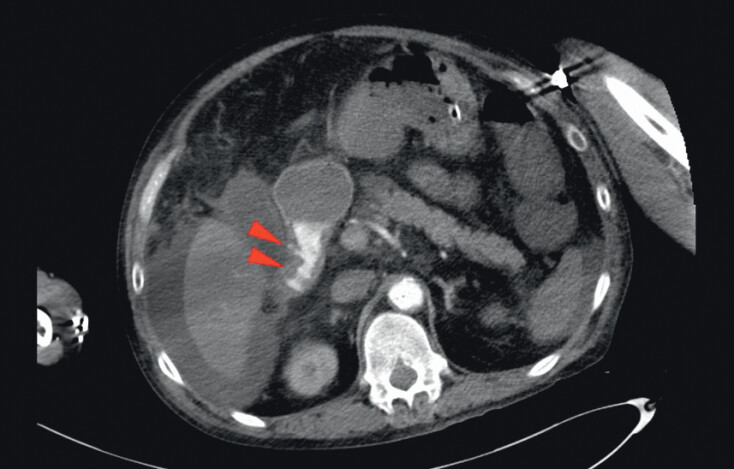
Contrast-enhanced computed tomography obtained at the referring
